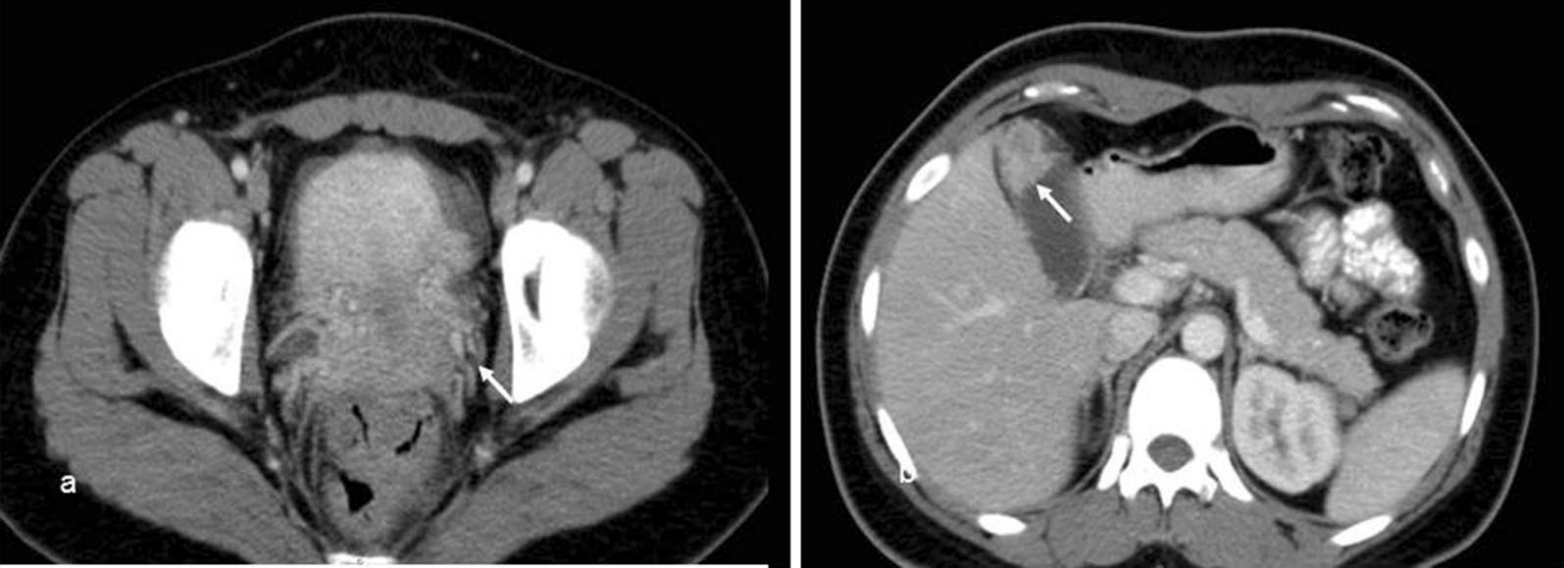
Contrast abdominal CT. a An irregular margin tumor located at right ovary (arrow). b A irregular tumor lesion enhanced and located at gallbladder fundus (arrow). But no symptom of extra-capsule invasion
